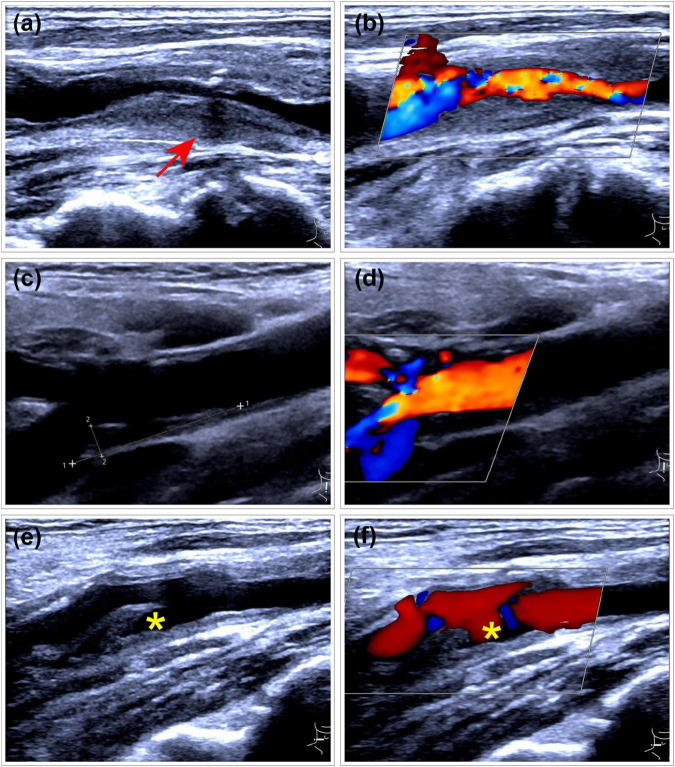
Representative ultrasound images of Plaque-RADS Category 3 subtypes (3A–3C). (a,b) RADS 3A plaque at the carotid bifurcation with a maximum wall thickness (MWT) greater than 3 mm. The plaque exhibits a uniform isoechoic appearance (arrow) and features a thick fibrous cap. (a) Gray-scale ultrasound imaging. (b) Doppler flow imaging. (c,d) RADS 3B plaque at the carotid bifurcation with a MWT greater than 3 mm. The plaque contains multiple very low echogenic areas, with most regions lacking a visible (thin) fibrous cap. (c) Gray-scale ultrasound imaging. (d) Doppler flow imaging. (e,f) RADS 3C plaque with a mixed hyperechoic and hypoechoic plaque at the carotid bifurcation, demonstrating ulceration (*) on both two-dimensional and Doppler imaging.
